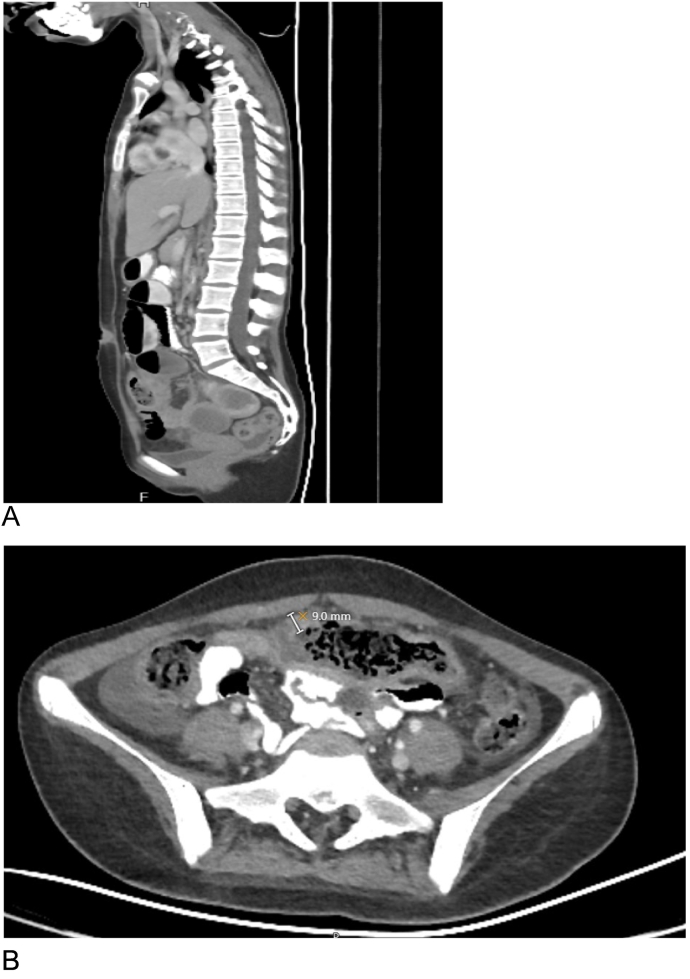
CT scan; sagittal view with oral contrast: shows multiple air-fluid levels within visualized small bowel loops. B: Axial CT scan with IV and oral contrast shows; increased transverse bowel wall thickness of around 1 cm.
